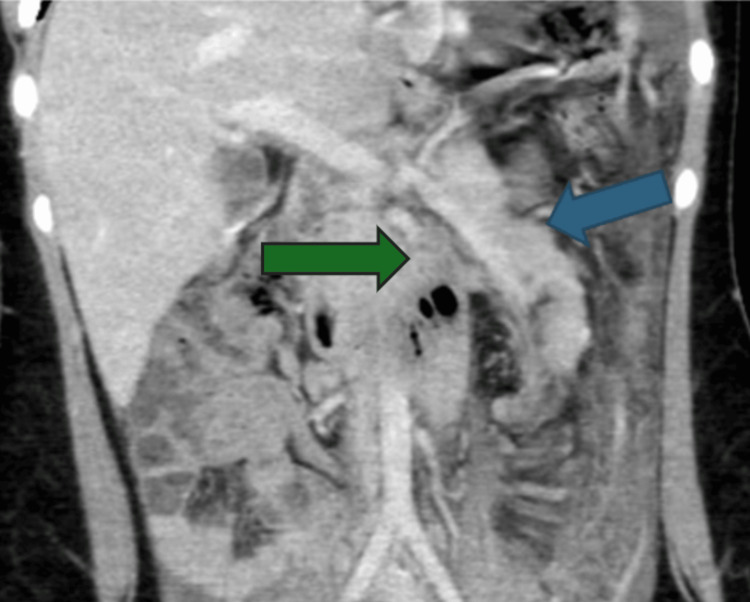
Contrast CT showing the ectopic spleen and the twisted splenic vascular pedicle (whirl sign) Green arrow: ectopic spleen; Blue arrow: splenic vascular pedicle
